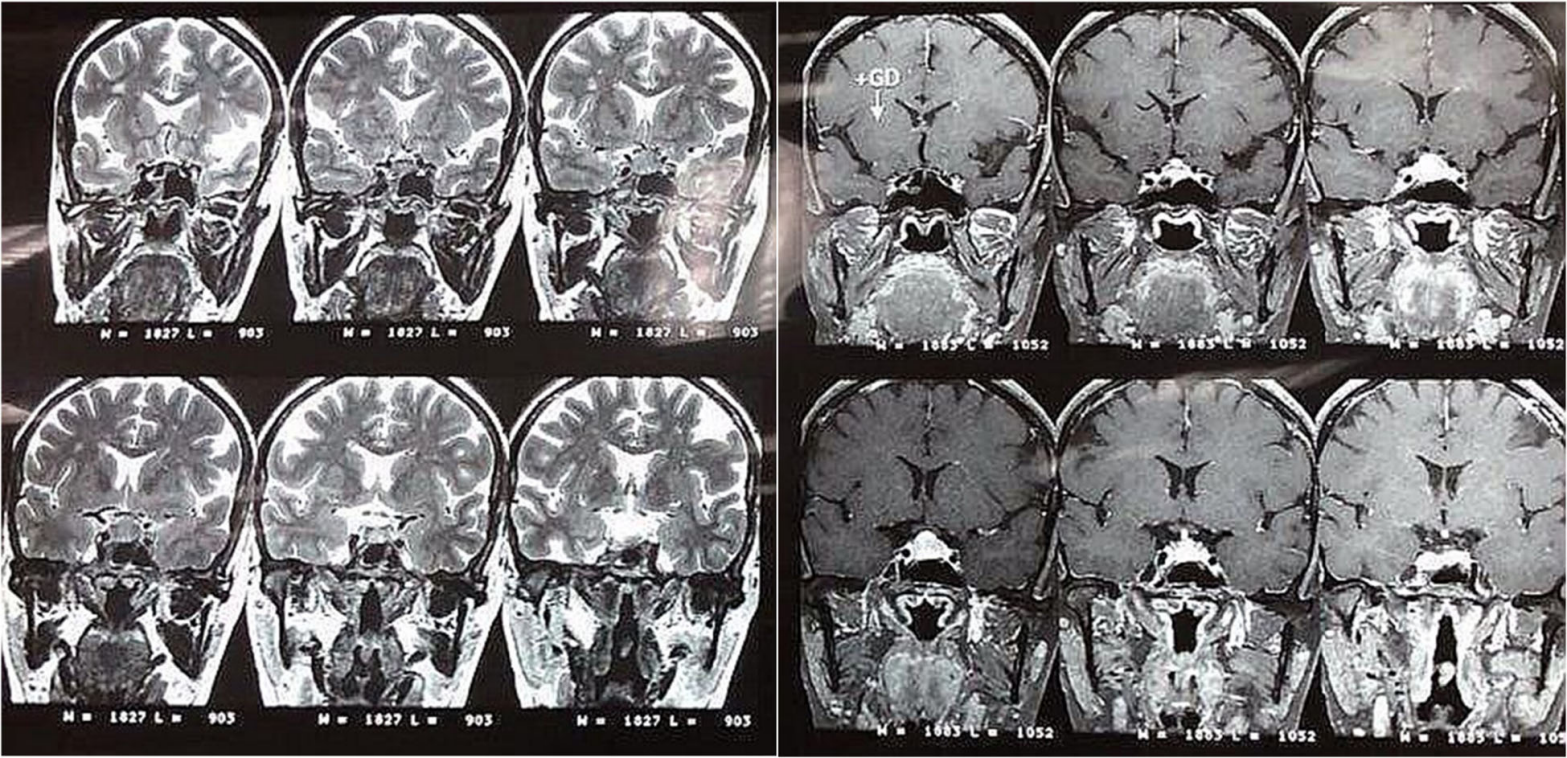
Pituitary magnetic resonance imaging without and with gadolinium enhancement showing an intermediate signal intensity mass of approximately 16 × 11 mm dimensions within the pituitary gland extending to suprasellar cistern causing mild mass effect on optic chiasma compatible with pituitary macroadenoma. Cavernous sinus appears normal and there is no evidence of invasion
